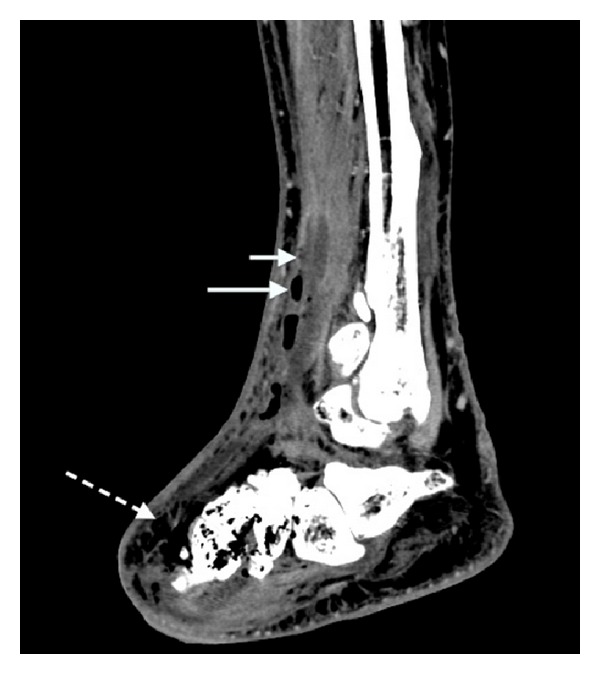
Computed tomography demonstrates soft tissue abscess formations anterior to the distal tibia with fluid (short arrow) and air-fluid levels (long arrow). Gas locules in the midfoot bones and extensive subcutaneous gas involving predominantly the dorsum of the foot (dashed arrow) and anterior aspect of the ankle are evident.
